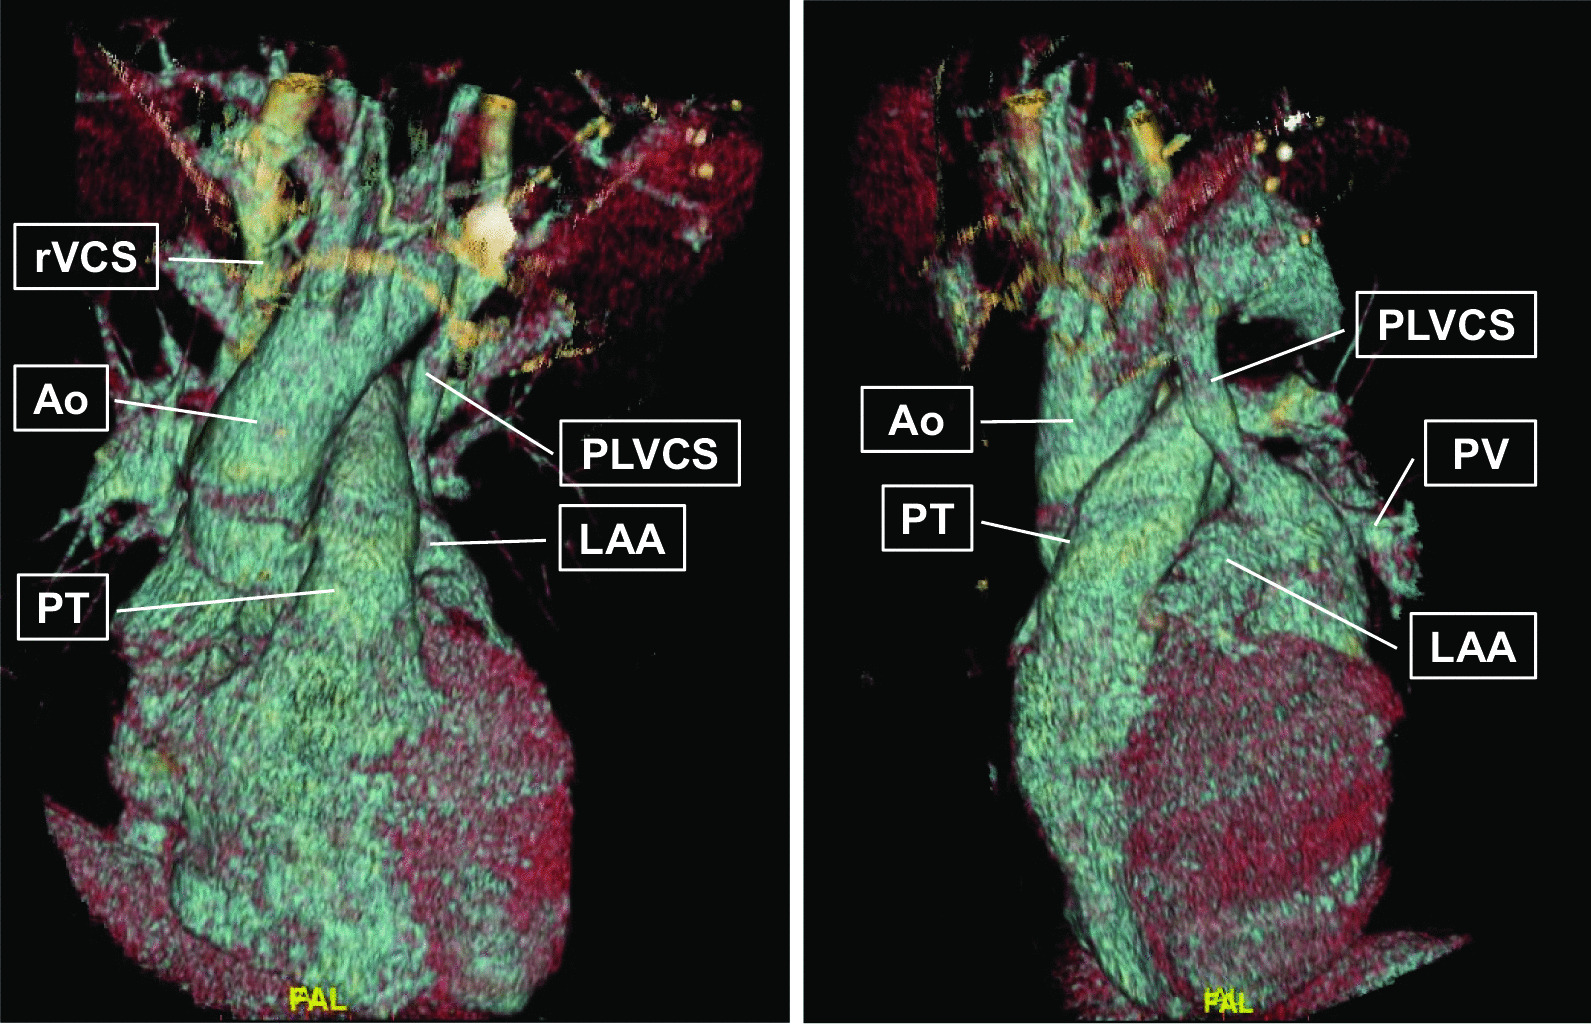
Reconstructed CT imaging reveals the atypical drainage of the persistent left vena cava superior (PLVCS) into the left atrial appendage (LAA). The lateral view (left image) exposes a more precise view this rare anatomical variant, while the anterior view (left image) displays the bilateral superior caval vein (*rVCS* right vena cava superior). *Ao* Aorta, *PT* Pulmonary truncus
